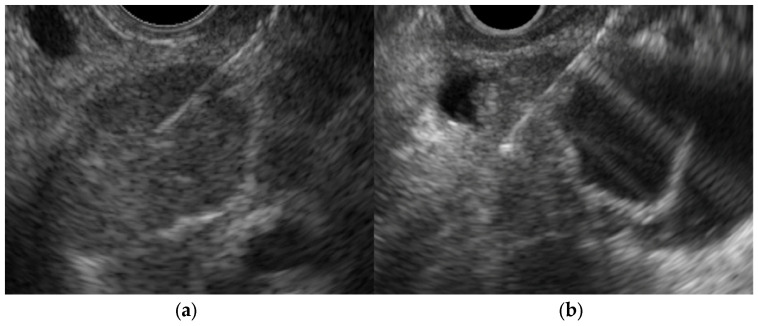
Endoscopic ultrasound-guided fine-needle aspiration/biopsy for malignant GB lesion. (a) Puncture of the enlarged lymph node. (b) Puncture of the GB wall-thickening lesions.
